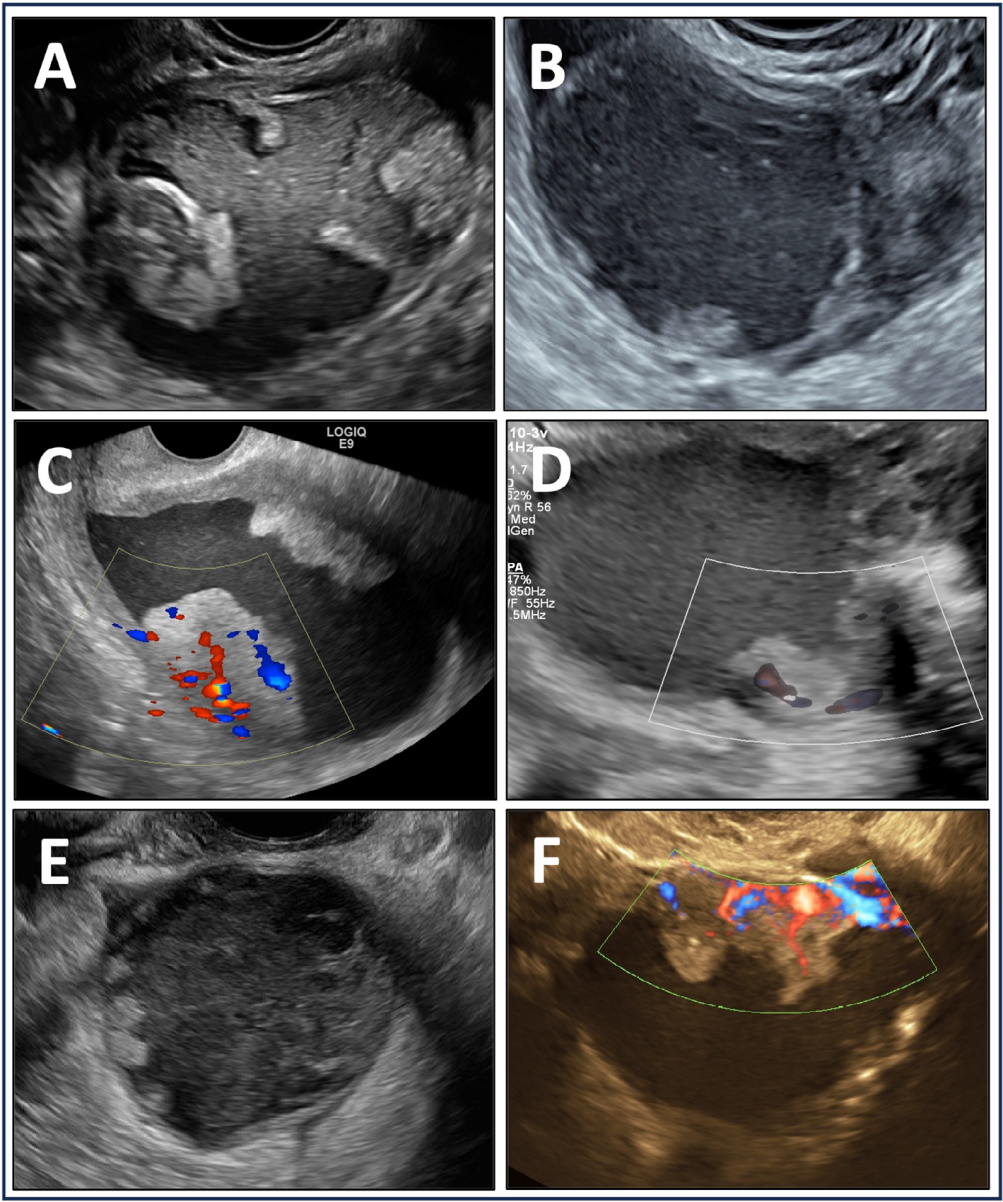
Seromucinous borderline ovarian tumors and differential diagnosis. Seromucinous borderline ovarian tumors are often cystic lesions with numerous vascularized papillary projections and ground‐glass cyst echogenicity, reflecting their association with endometriosis (A–D). Notably, in the differential diagnosis, atypical endometrioma may present as a cystic lesion with ground‐glass cyst echogenicity and papillary projections; however, it usually does not show vascular flow on Doppler imaging (E). In contrast, decidualized endometriomas during pregnancy may also contain papillary projections that are almost always highly vascularized on Doppler imaging (F).
